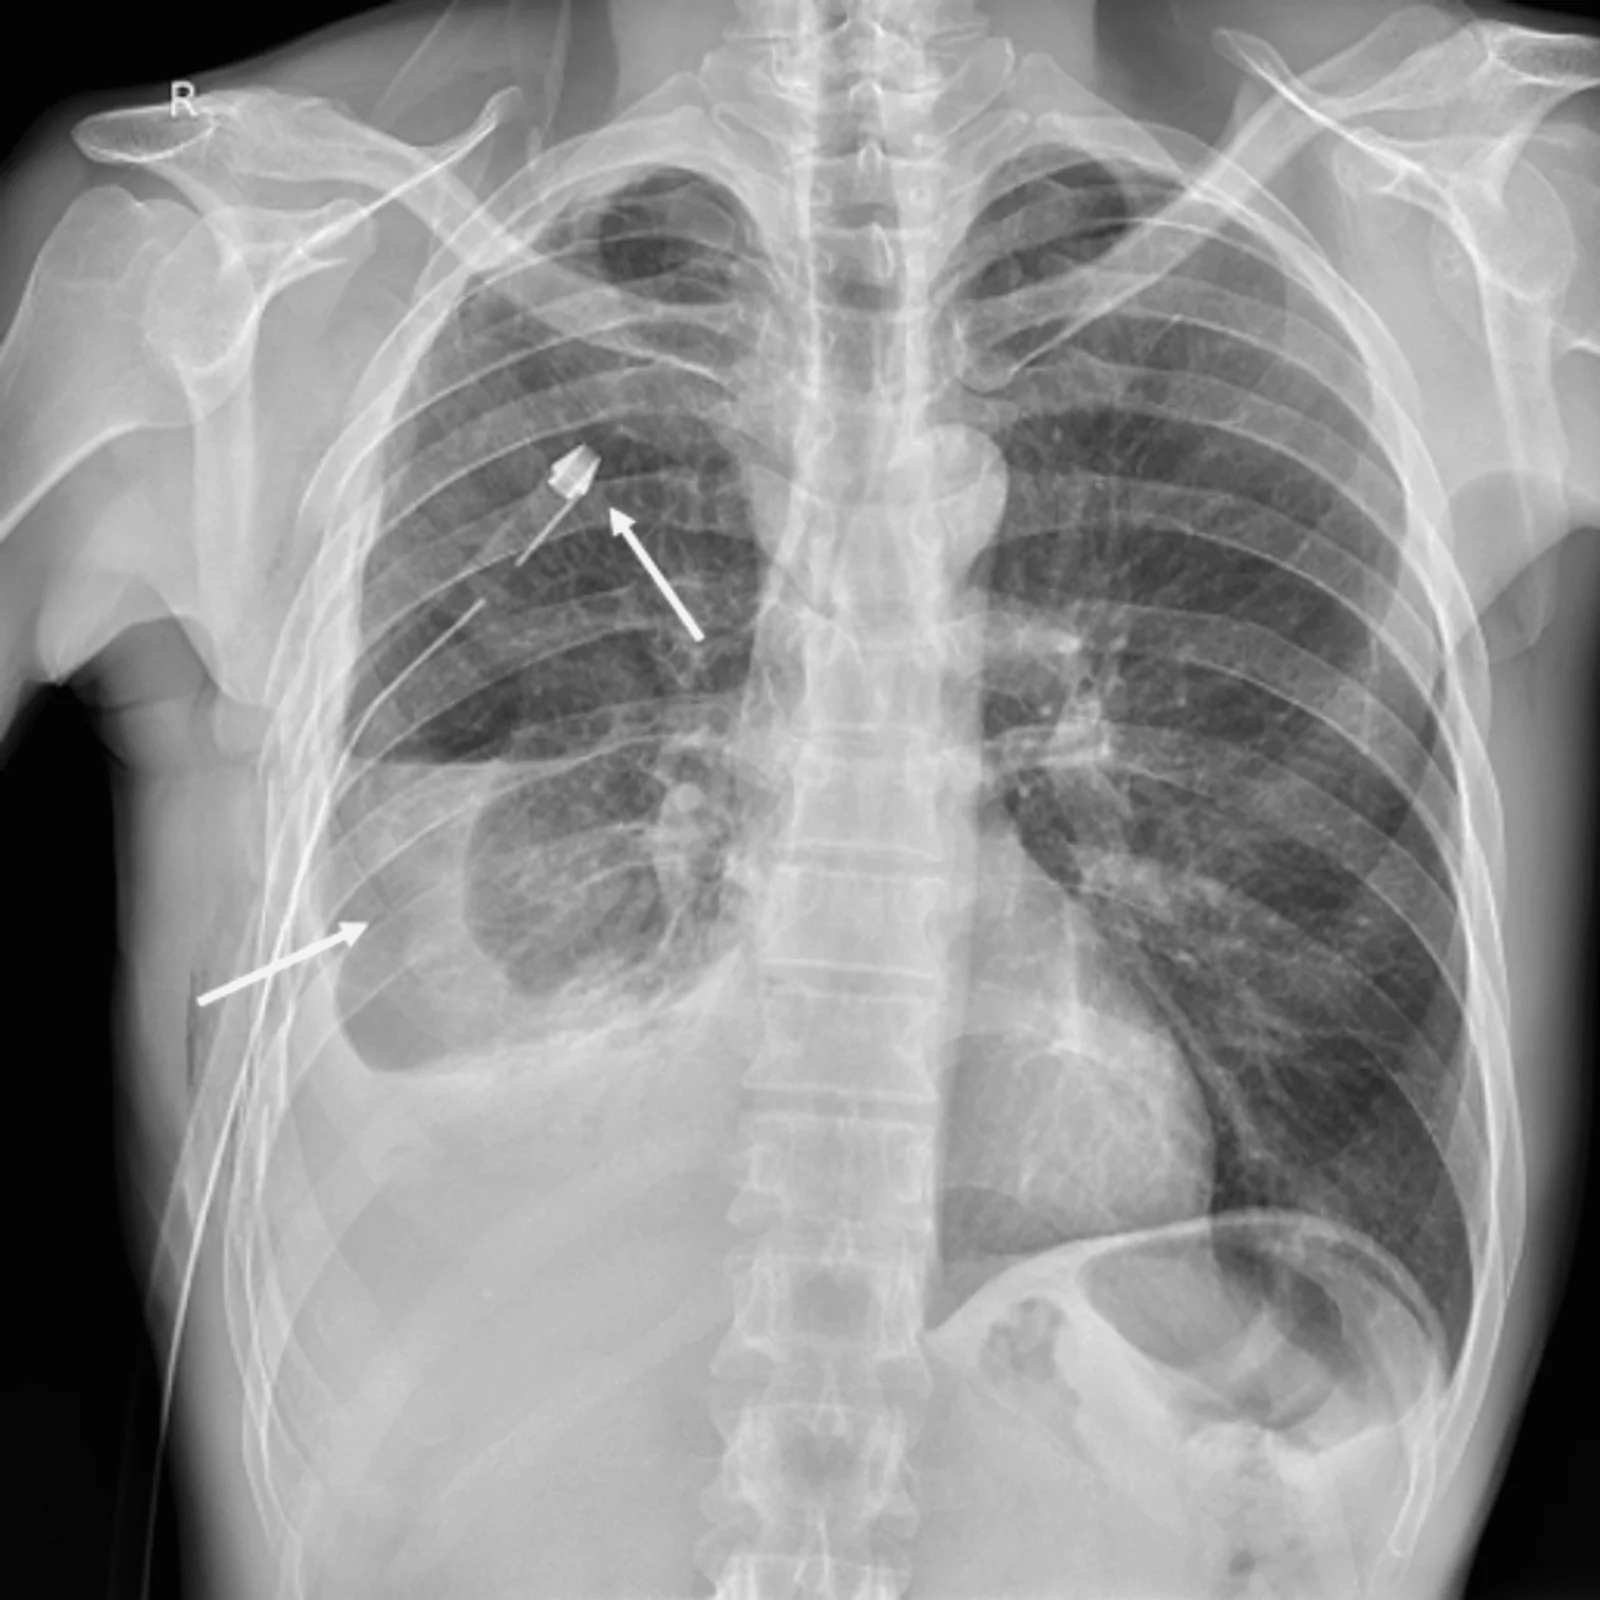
Post-intervention anteroposterior chest radiograph on day one of admission. The image demonstrates a newly inserted right-sided chest tube positioned within the upper pleural space (upper arrow). Compared to the previous radiograph, a residual air-fluid interface of the resolving hemopneumothorax is visible (lower arrow), demonstrating significant volume reduction and substantial re-expansion of the right lung parenchyma.
